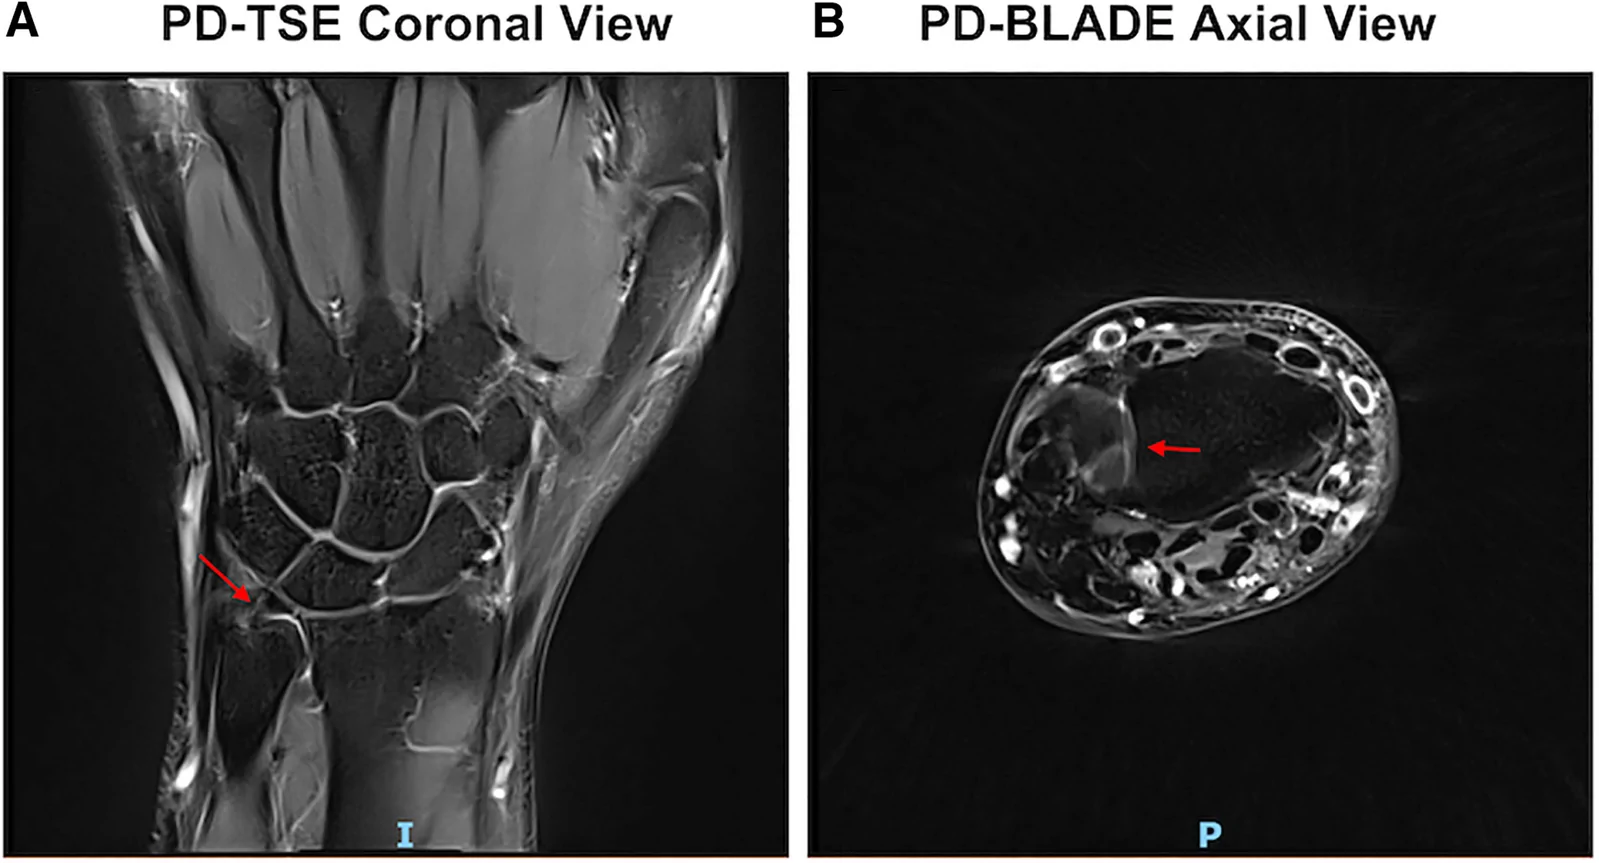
Magnetic resonance imaging of the case 2. (A) Coronal view demonstrating moderate-to-long signal intensity extending from the upper disc surface to the bottom, indicating a possible chronic TFCC injury (red arrow); (B) moderate-to-long signal runs diagonally through the disc (red arrow), consistent with the morphology in the coronal view. TFCC = triangular fibrocartilage complex.
